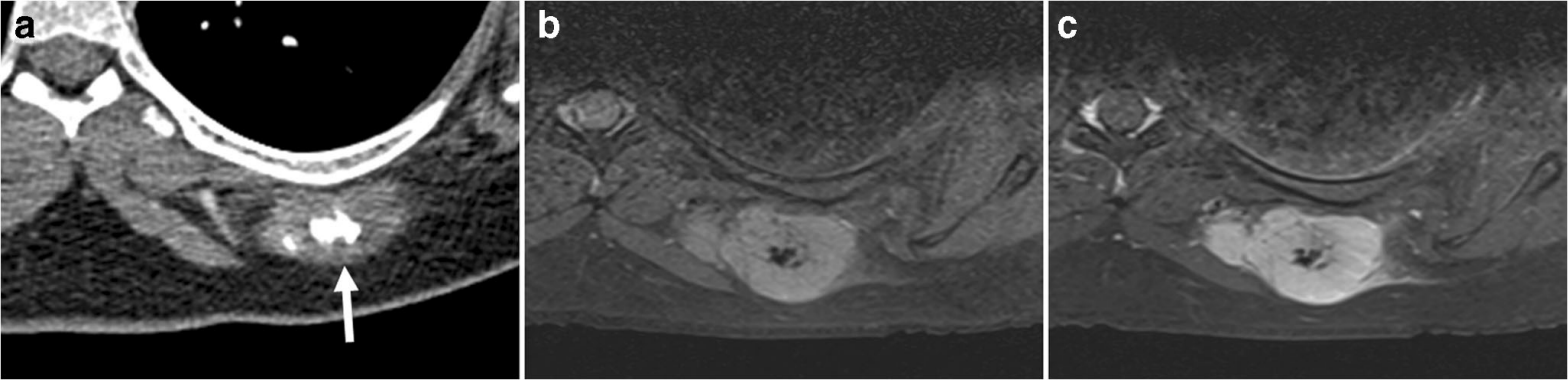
A 22-year-old woman with Castleman disease. a Axial CT shows a well-defined subcutaneous soft tissue mass with central calcification at the left thoracic region. b, c Axial pre- (b) and postgadolinium (c) T1-weighted images with fat suppression show homogeneous enhancement of the mass (except for the central calcification). The mass was biopsied and pathologically proven to be Castleman disease
